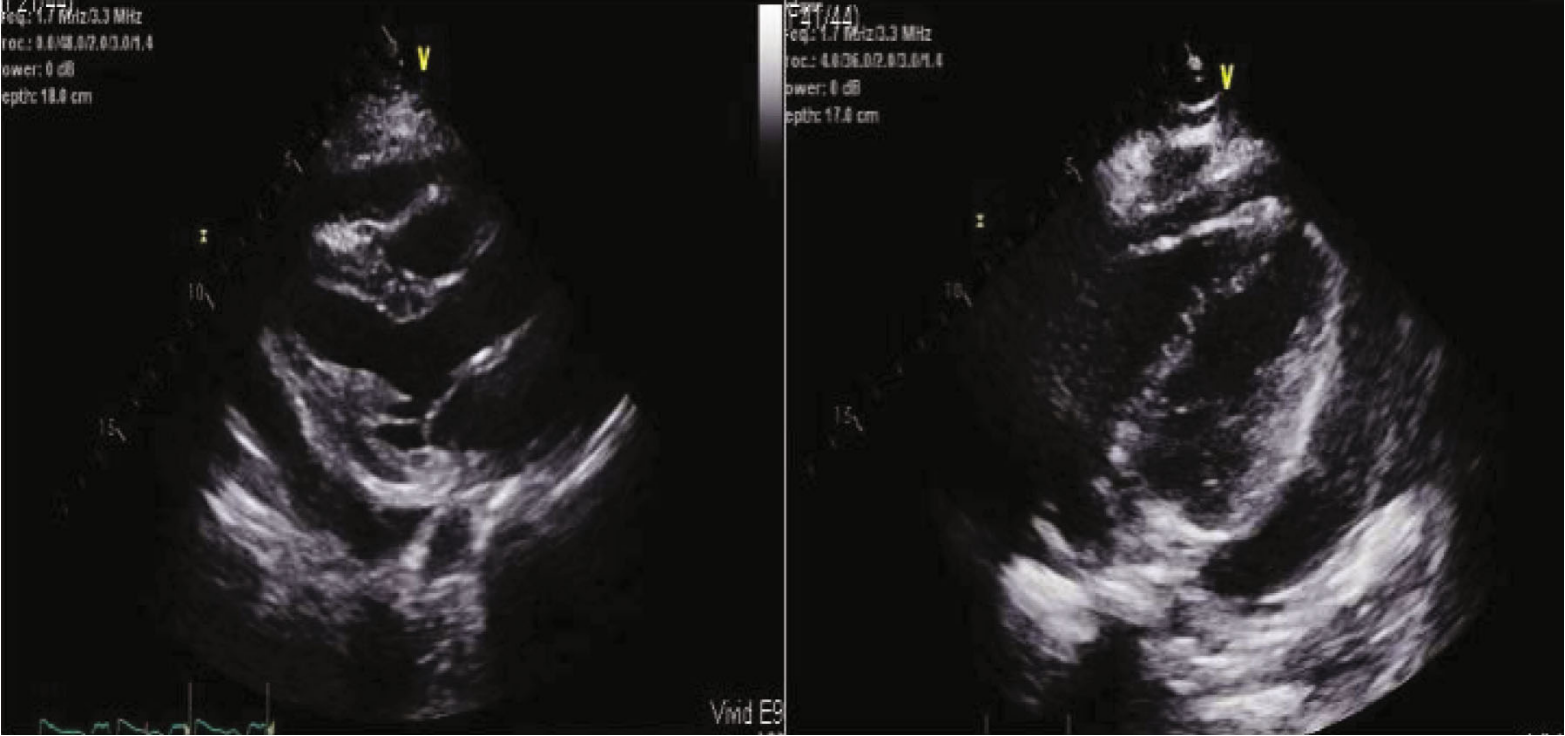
Echocardiogram images demonstrating a large pericardial effusion. Cardiac tamponade physiology was present during the study.
